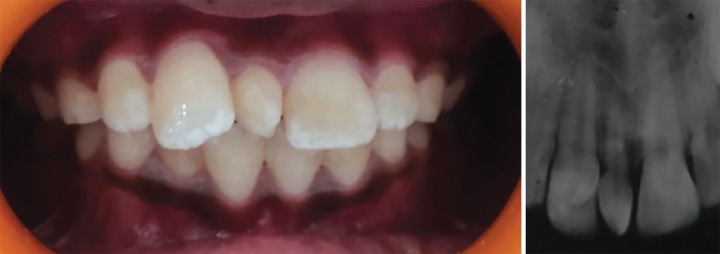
Pretreatment view showing mesiodens: (A) Teeth front view; and (B) IOPA radiograph
